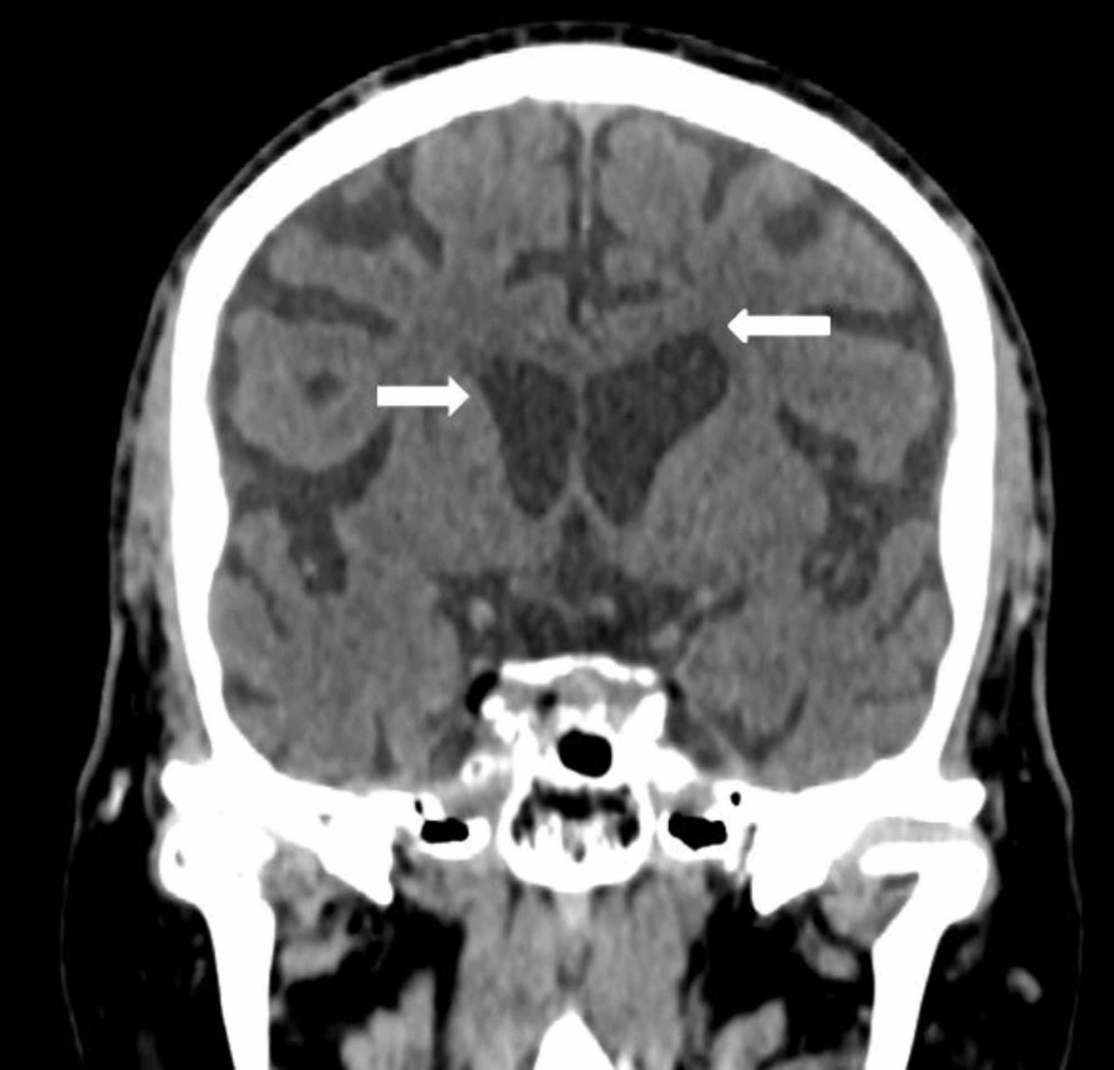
A selected coronal section of the unenhanced CT scan of the head demonstrating prominence of the ventricles (white arrows) and age-related involutional changes
